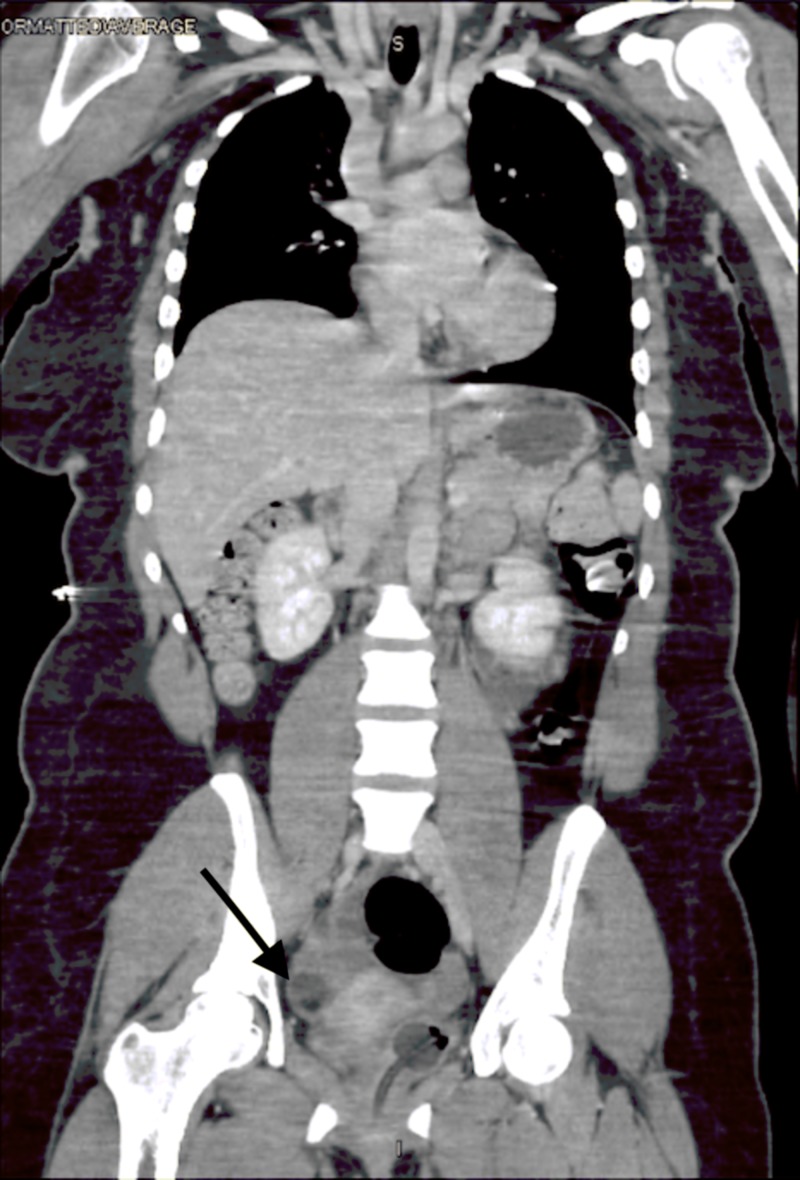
Coronal CT Chest Abdomen and Pelvis with IV Contrast
